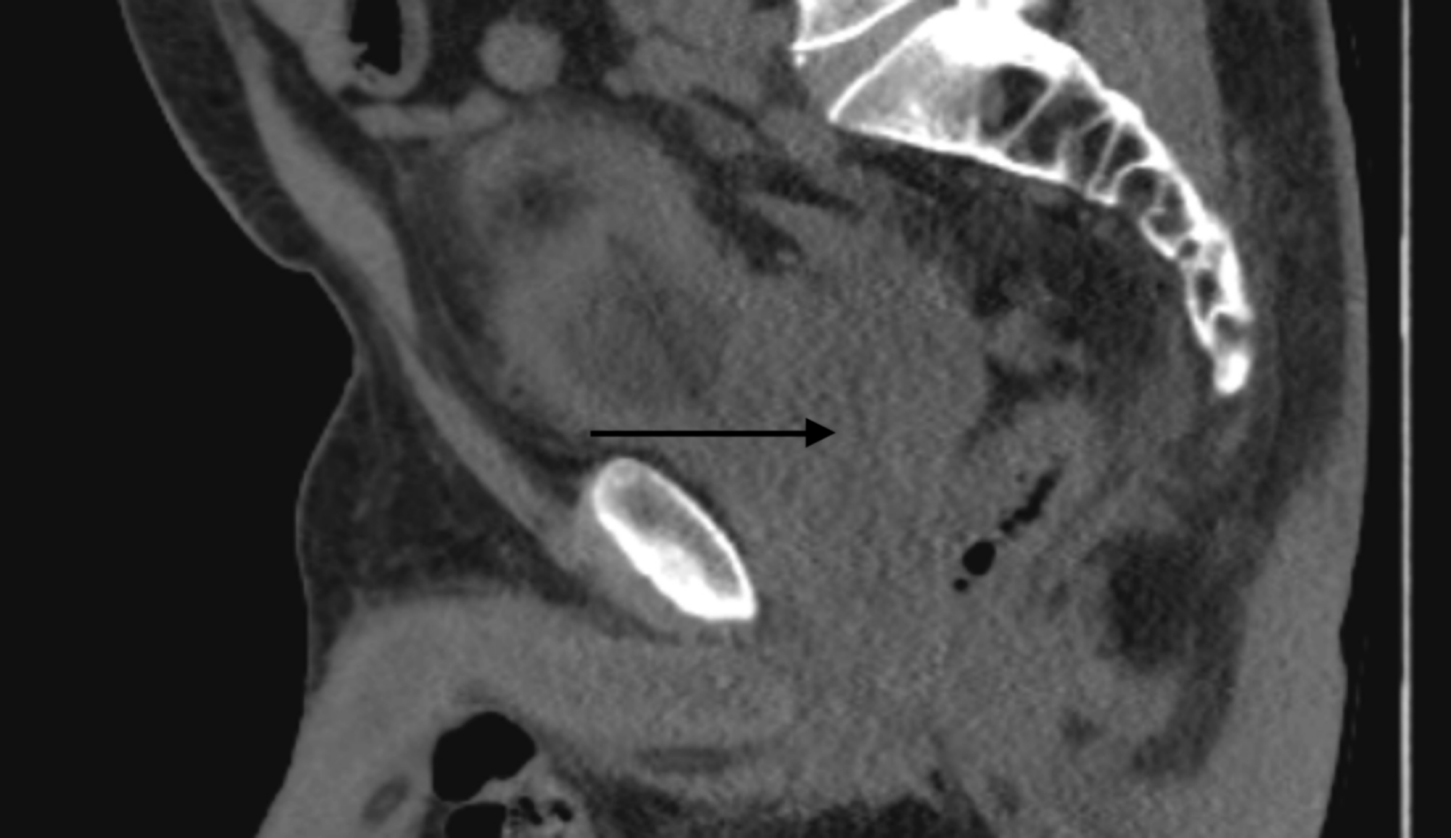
Sagital view of the CT pelvis Black arrow points toward the prostate
